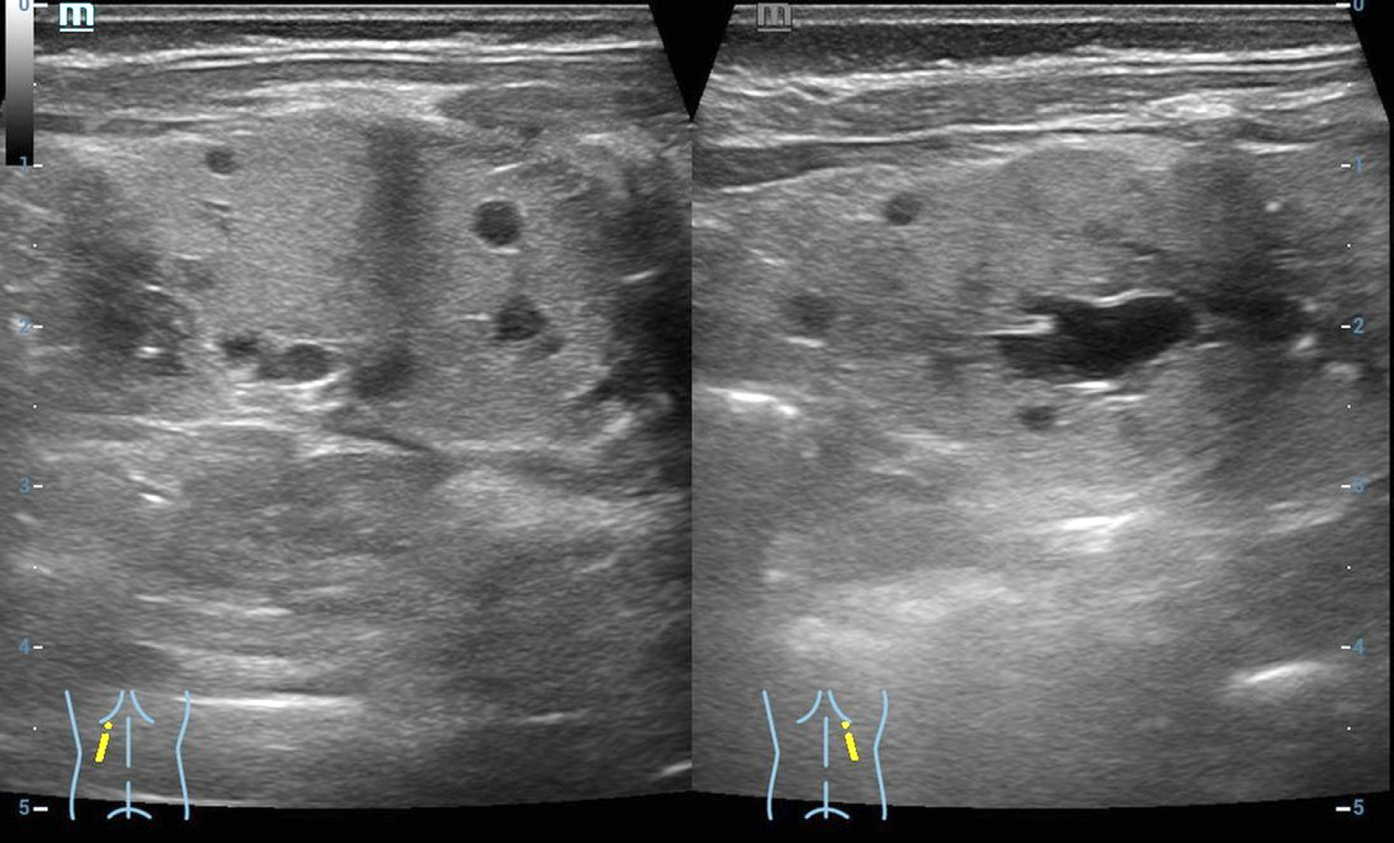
Patient kidney ultrasound. Increased echogenicity of the renal parenchyma on both sides, poor corticomedullary differentiation, thickened parenchyma, small multiple cysts in the parenchyma from immeasurable to 0.6 cm. The size of both kidneys 5 × 2.1 cm.
